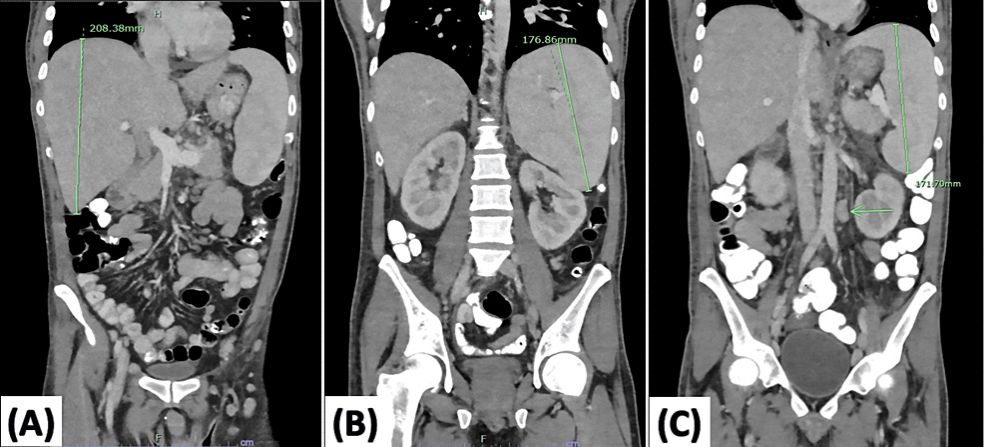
Coronal views of computed tomography (CT) of the chest, abdomen, and pelvis with contrast were completed during the first hospital encounter. (A) New hepatomegaly in the right lobe measuring 21 cm (previously 16 cm). (B) New splenomegaly measuring 17.7 cm (previously 8.9 cm) and bilateral nephromegaly (measurements not shown; left kidney 12.9 cm and right kidney 12.6 cm, both previously 11.3 cm). (C) Para-aortic lymphadenopathy (indicated by the green arrow, measuring >2 cm).
